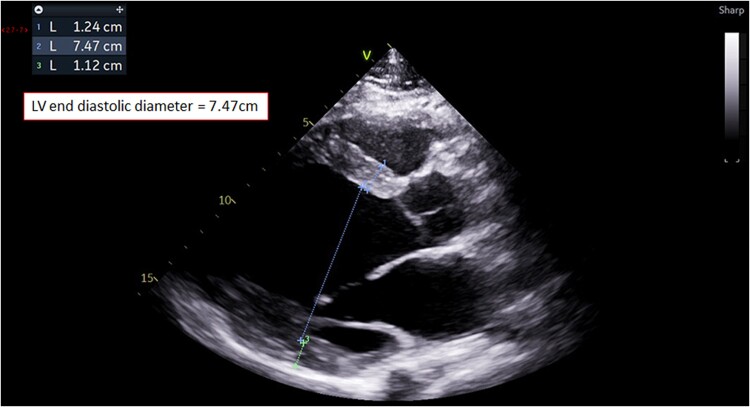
Transthoracic echocardiography—left ventricle diastolic dimensions.
